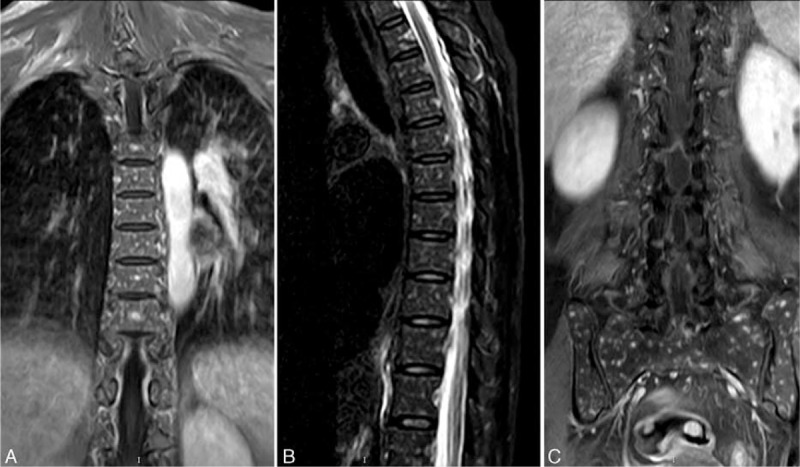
MRI of the thoraco-lumbar spine. Coronal STIR sequences (A), axial T2WI (B), and coronal STIR sequences (C) demonstrated extensive high signal in the thoracic vertebrae, sacrum and ilium, showing hematogenous disseminated miliary lesions. MRI = magnetic resonance Imaging, STIR = short-tau inversion recovery.
